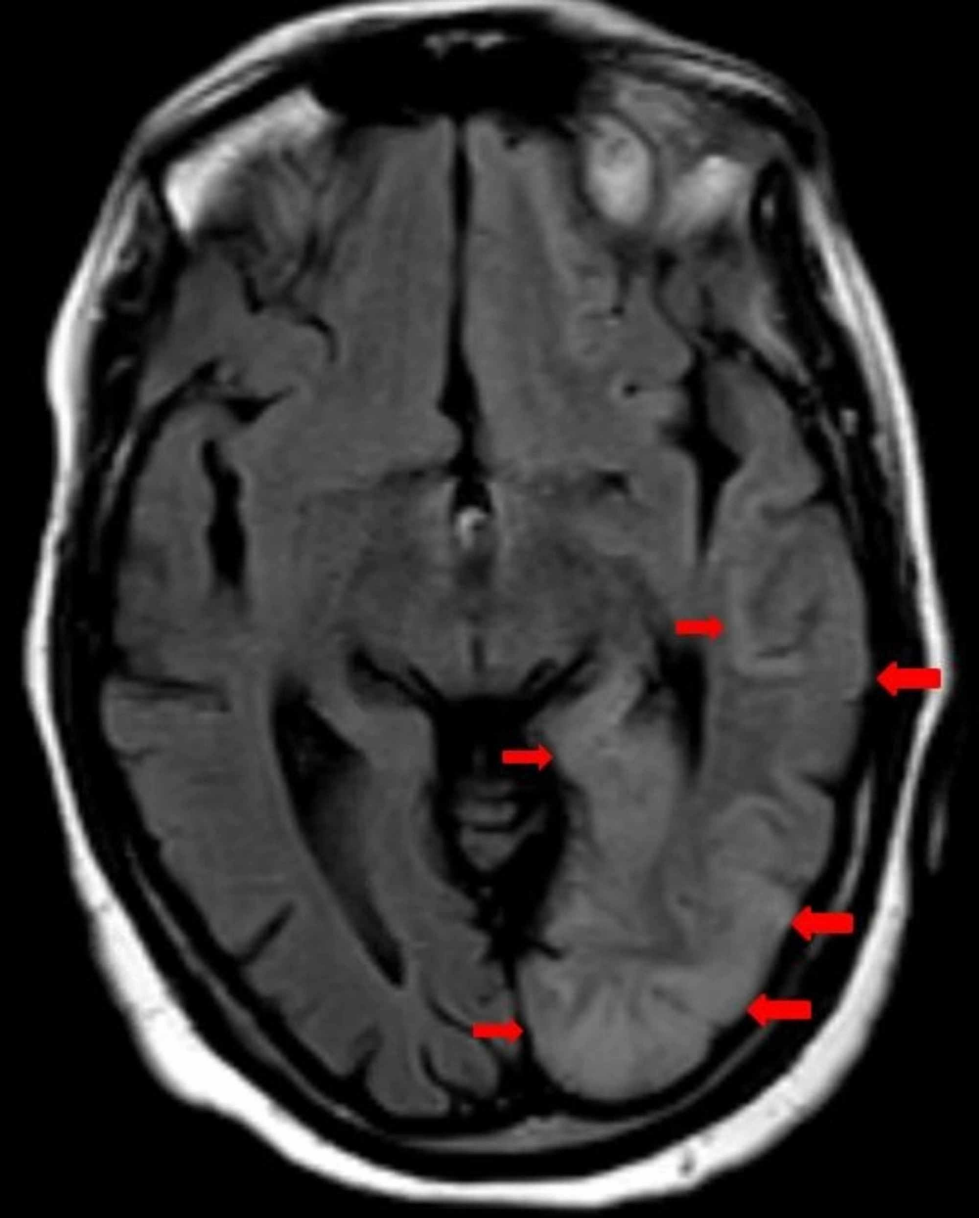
A selected axial T2-weighted FLAIR image It is showing slightly high signal intensity (red arrows) in the left temporal, parietal, and occipital regions. These signals are diffusely involving the white and grey matter. FLAIR: fluid-attenuated inversion recovery.
